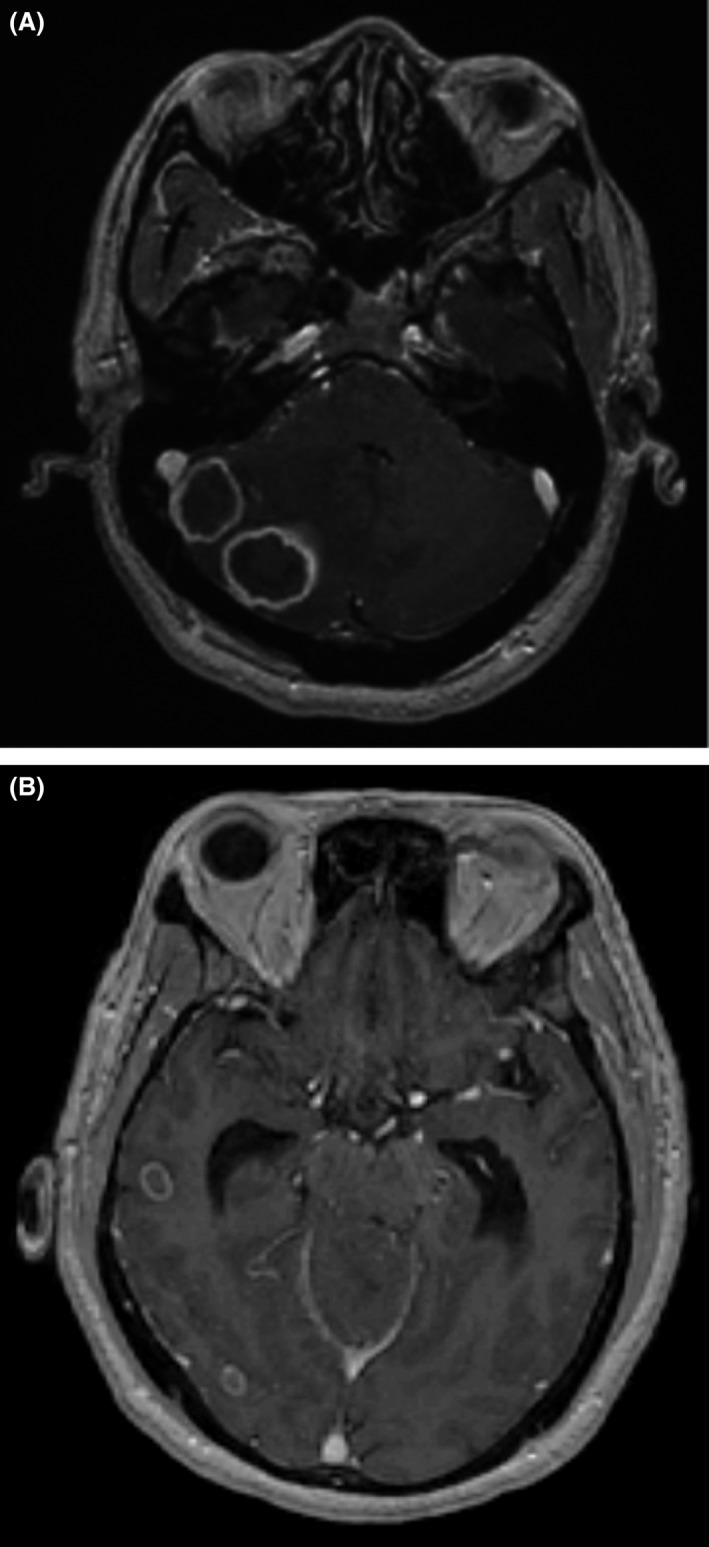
3D Fast Spoiled Gradient‐Recalled‐Echo MRI demonstrating infratentorial ring‐enhancing lesions in the right cerebellum with surrounding vasogenic edema and consequential partial effacement of the fourth ventricle (A), as well as additional lesions in the right temporal lobe (B).
